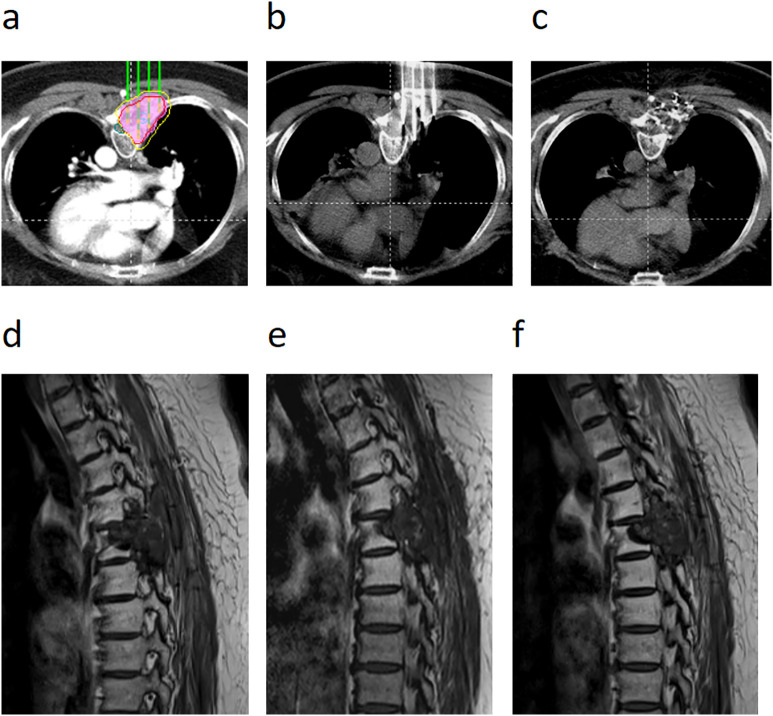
A patient with thoracic spine metastasis after resection of lung cancer. (a) Under preoperative enhanced CT guidance, the ^125^I seed implantation system was used for preoperative planning. (b) In interventional implantation, imaging after the template-assisted CT-guided needle insertion showed that the needle insertion angle and the depth were good, and the large blood vessels were successfully avoided. (c) Immediate post-interventional review; CT revealed a uniform seed distribution in the lesion area. (d) Preoperative MRI showed space-occupying lesions in the 4th and 5th thoracic accessory areas as well as the posterior costal region. (e) MRI at 1 year after seed implantation showed that the lesion had narrowed. (f) MRI 2 years after intervention showed that the boundary of the lesion was clear and the lesion was stable.
